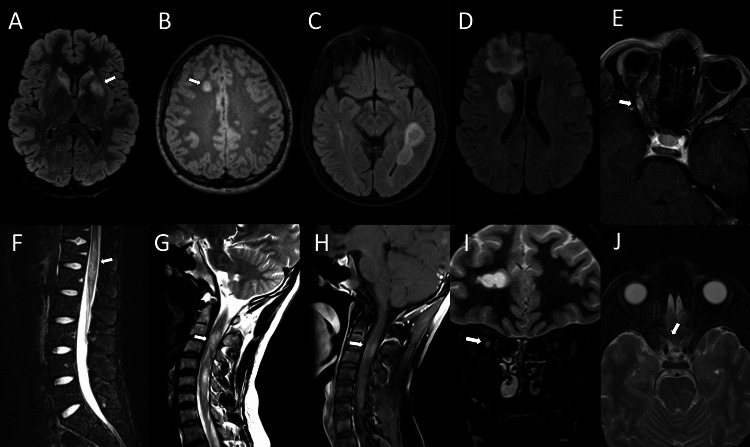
MRI brain and spine from Case 2 at initial presentation to our service. FLAIR MRI, axial view, showing T2-hyperintense lesions involving left caudate (A), right frontal corona radiata (B), left temporal lobe (C), right frontal lobe (D), and right optic nerve sheath (E). T2/short-tau inversion recovery MRI, sagittal view, showing T2-hyperintense conus medullaris (F). Sagittal cervical spinal cord with T2-hyperintense signal (G), associated with enhancing signal on sagittal T1 post-contrast (H). T2 MRI, coronal view, showing right optic nerve hyperintense rim (I). T2 MRI, axial view, showing T2 hyperintensity on right optic nerve sheath (J). MRI: magnetic resonance imaging; FLAIR: fluid-attenuated inversion recovery image
